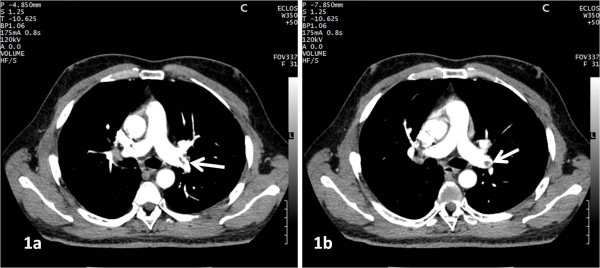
A 41-year-old man, with dyspnea of sudden onset. CT angiogram shows a typical embolus in the left pulmonary artery (arrow). No thromboembolic risk factor was found.
